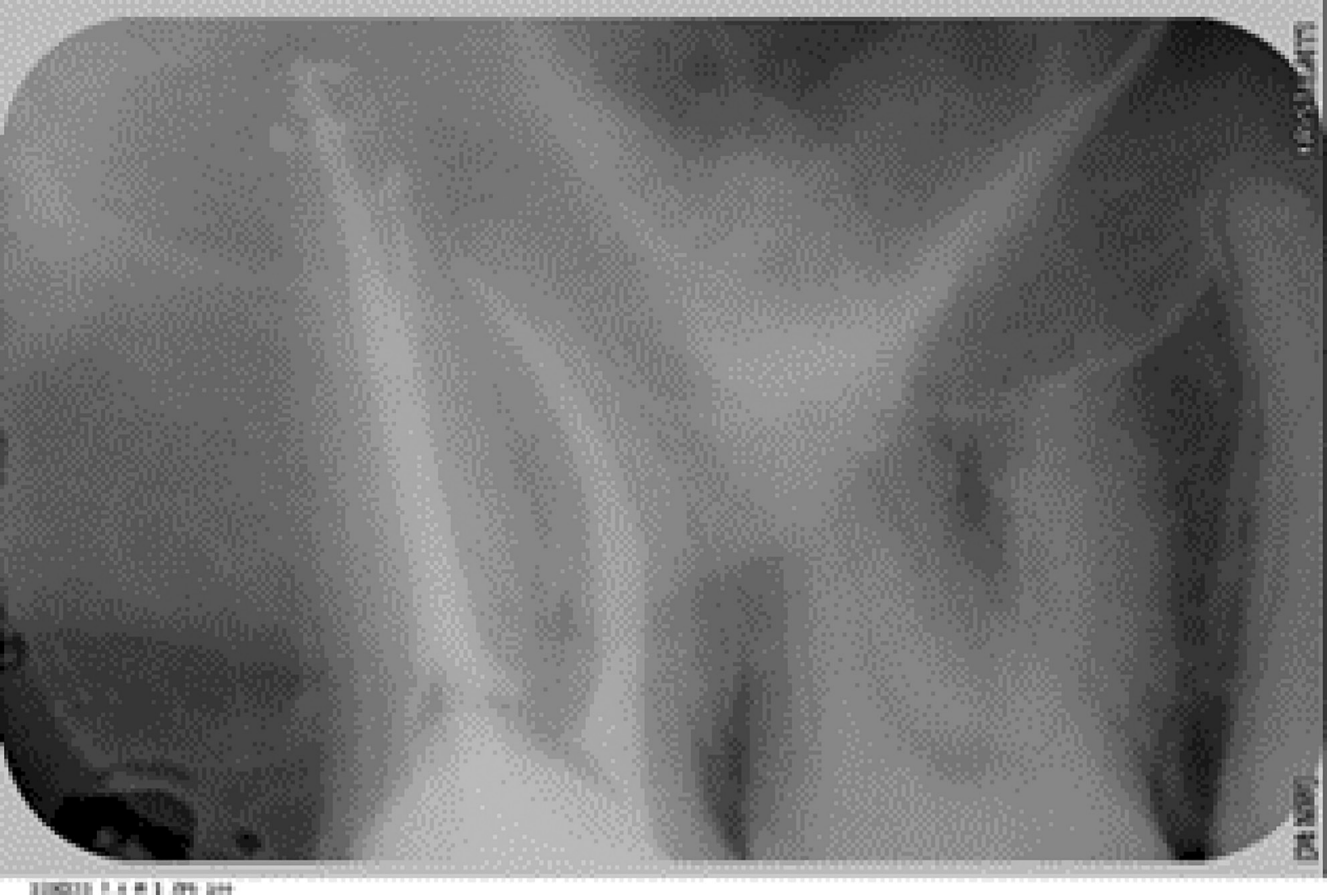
PA radiograph following root canal retreatment, tooth 17.
